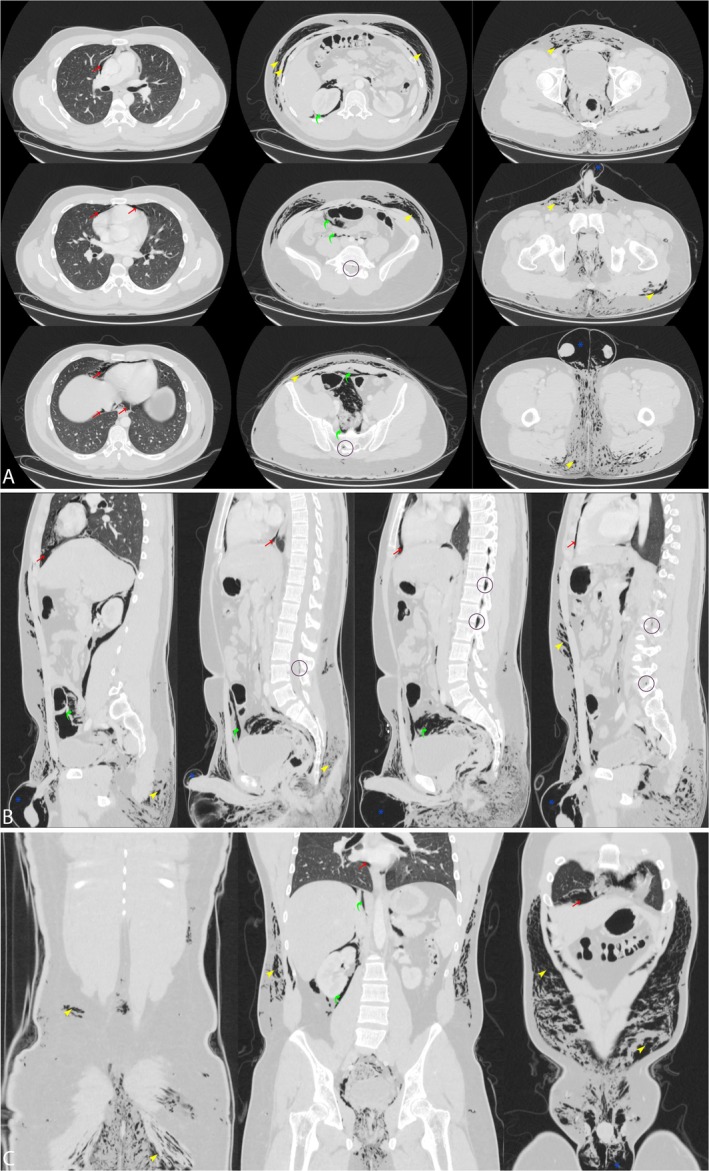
Computed tomography after perineal compressed‐air injury. Axial (A), sagittal (B), and coronal (C) images demonstrate extensive extraluminal air across multiple compartments. Air is present within the peritoneal and retroperitoneal spaces (green curved arrows), along the abdominopelvic fascial planes, and within the subcutaneous tissues of the chest wall, abdominal wall, and perineum (yellow arrowheads), as well as within the scrotum and penis (dark‐blue asterisks). Intraspinal air is also seen, consistent with pneumorrhachis, extending from approximately the T9 level caudally through the thoracolumbar and lumbosacral spinal canal, best appreciated on sagittal images (purple circles). Red arrows indicate air within the mediastinum, pericardial space, and bilateral pleural spaces. No definite persistent full‐thickness rectal wall defect or gross fecal contamination is evident on routine CT. The pattern is most consistent with widespread air dissection following an occult sub‐endoscopic anorectal mucosal breach, sealed luminal communication, or transient microperforation.
